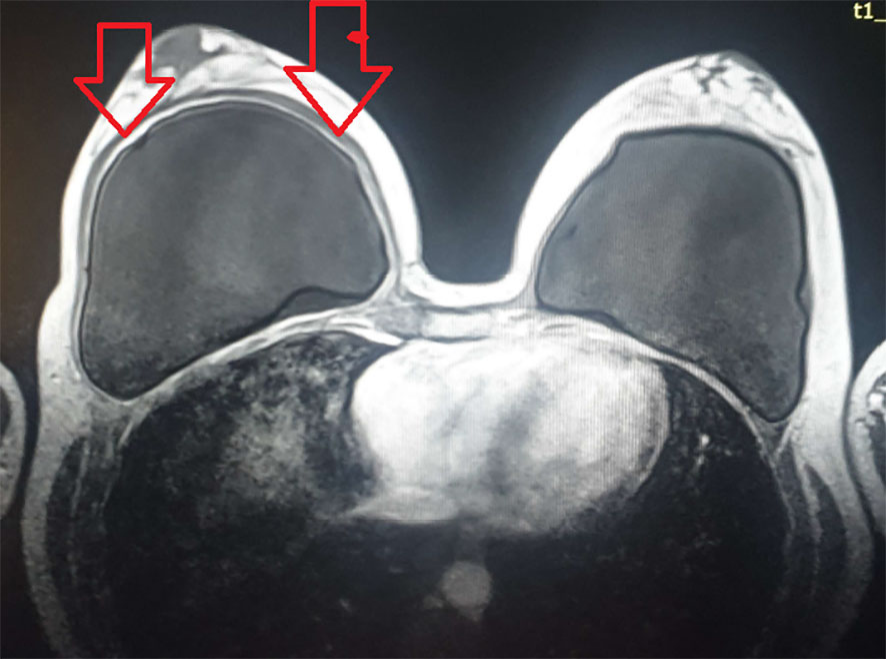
MRI after a silicone breast implant (SBI) showing the signs of right breast capsulitis. MRI, magnetic resonance imaging; SBI, silicone breast implant.
